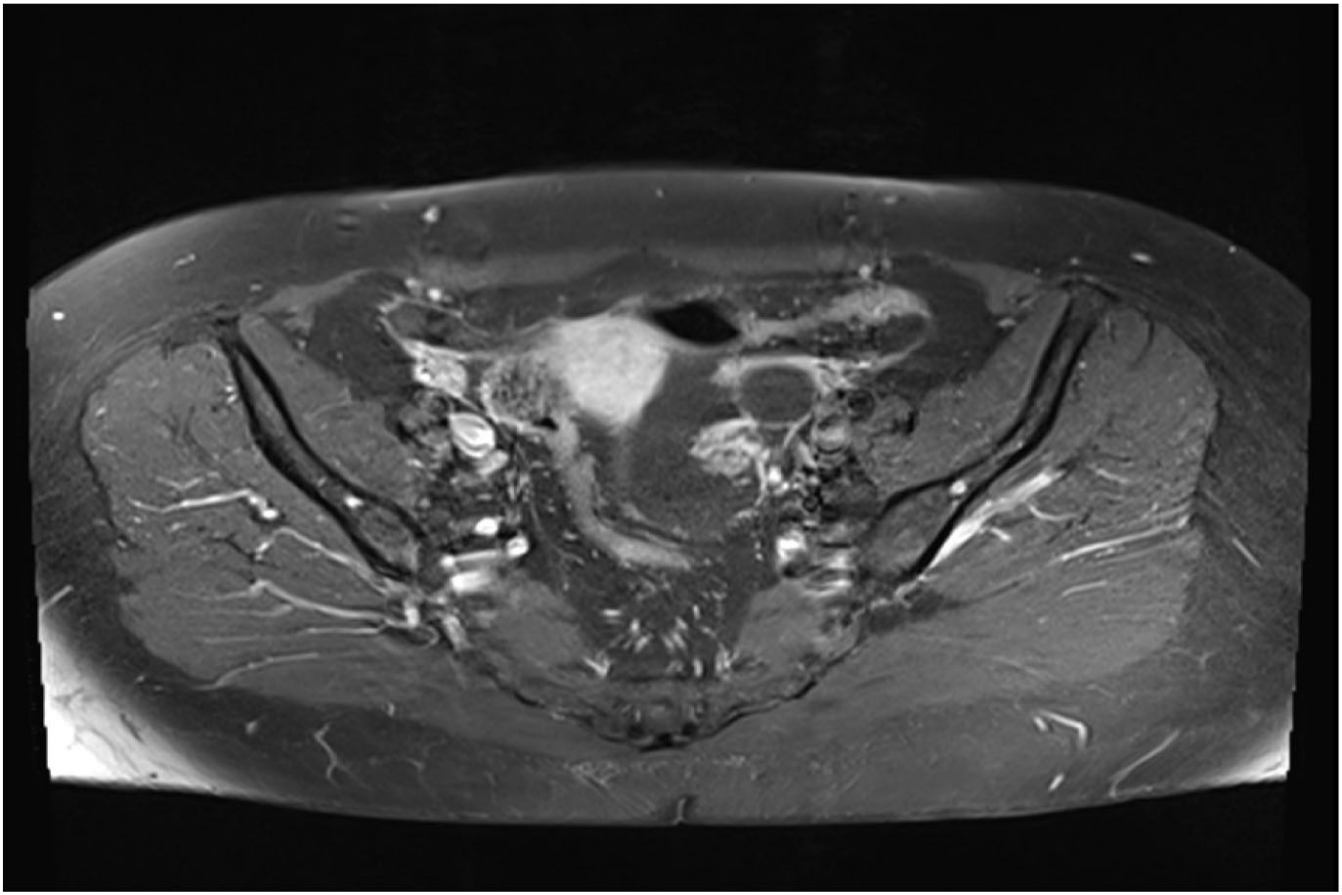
CT view of abdominal scan showing that the left ovary is independent of the effusion.
